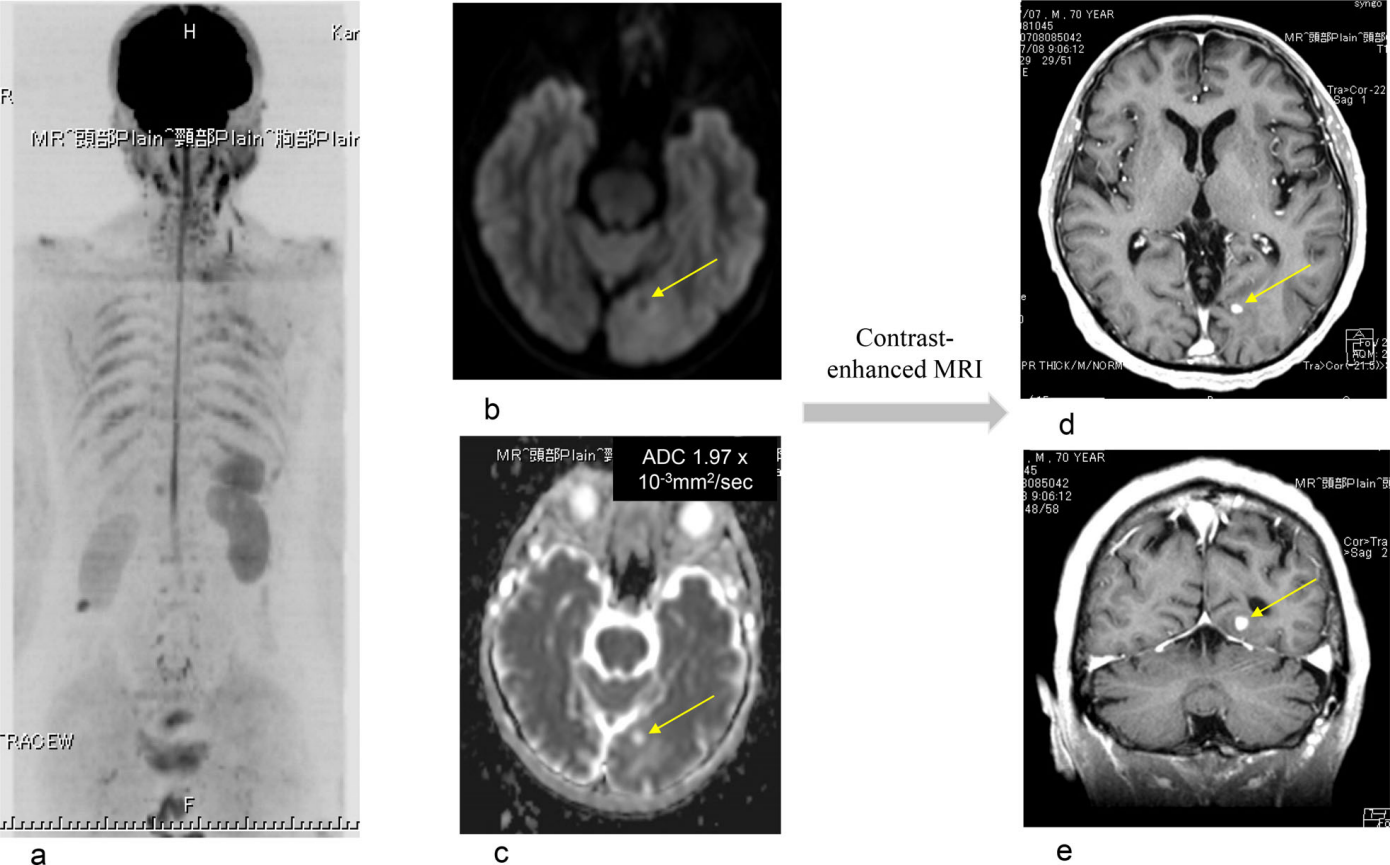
Case 4. Squamous cell carcinoma. pT3N0M0. (a) Whole body imaging in diffusion‐weighted whole‐body imaging with background suppression (DWIBS). (b) A tiny brain metastasis was presented in DWIBS. (c) The apparent diffusion coefficient (ADC) of the brain metastasis in the ADC map was 1.94 × 10^−3^ mm^2^/s, and was a false negative. (d, e) Contrast‐enhanced magnetic resonance imaging (MRI) determined a brain metastasis. The DWIBS‐enhanced lesion (ADC 2.04 × 10^−3^ mm^2^/s) at the level of the right kidney which was located in the abdominal wall and had not changed for one year was diagnosed as a benign neurogenic tumor
